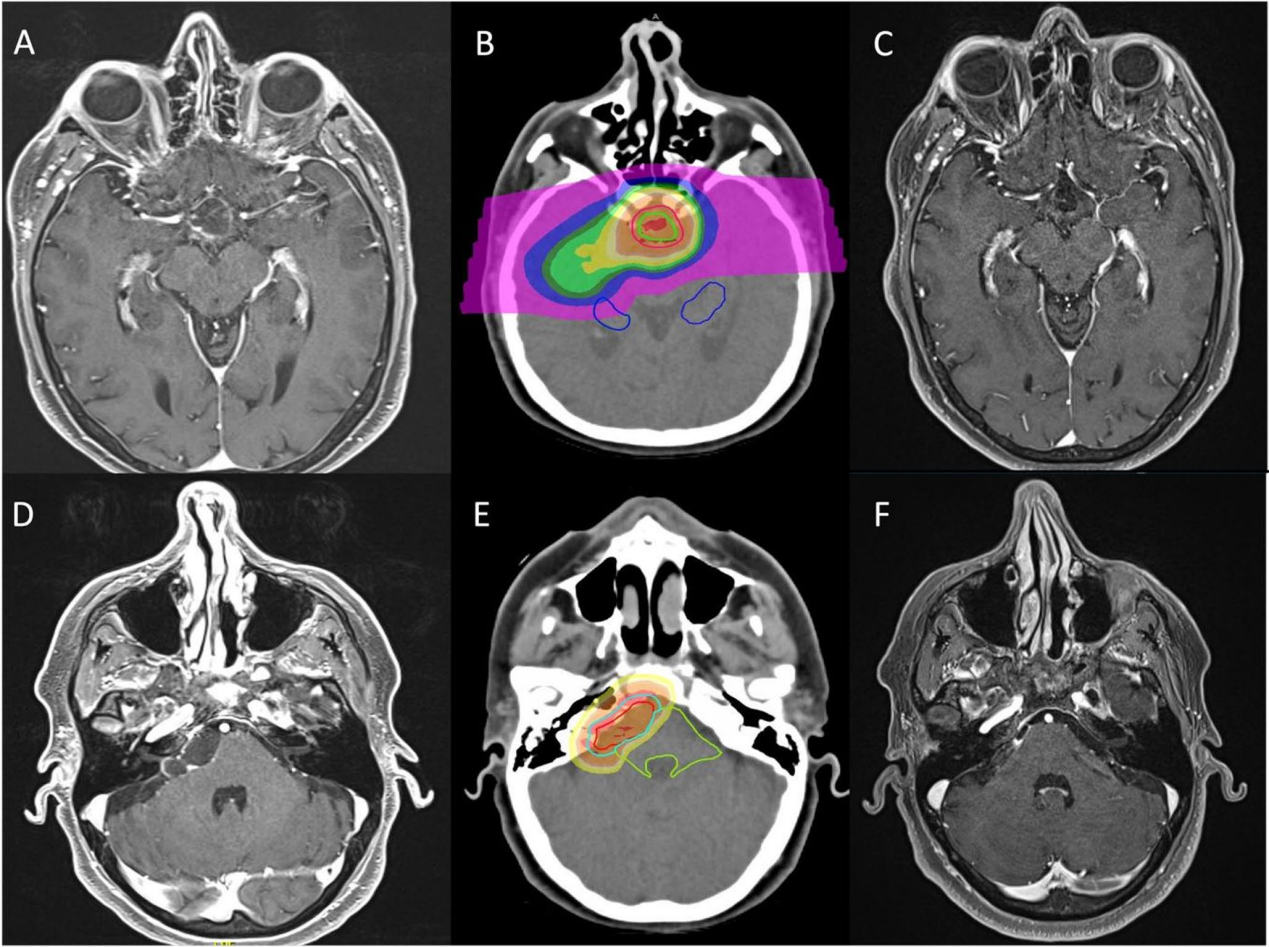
A, axial scan showing the recurrent cystic suprasellar component. B, PRT plan with hippocampi dose constraint respected (less than 40% of hippocampi volume receiving 7.3 GyRBE). C, Two-year follow-up scan with partial response. D, Axial scan view of the right ponto-cerebellar angle (APC) location of cystic recurrence. E, PRT plan focused on the APC recurrence abutting to the brainstem. F, Two-year follow-up MRI showing partial response
